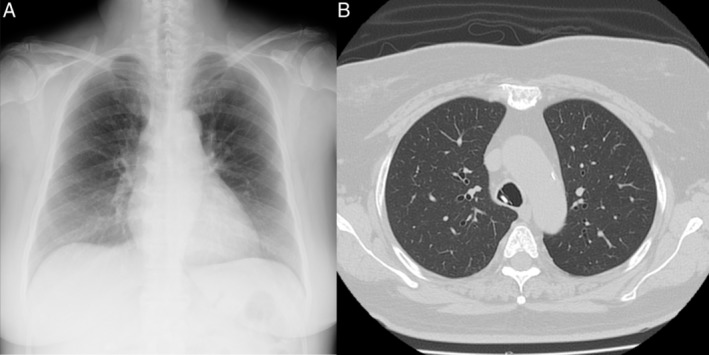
(A) Chest X‐ray showing no abnormal findings. (B) Computed tomography scan showing a foreign body in the trachea.
